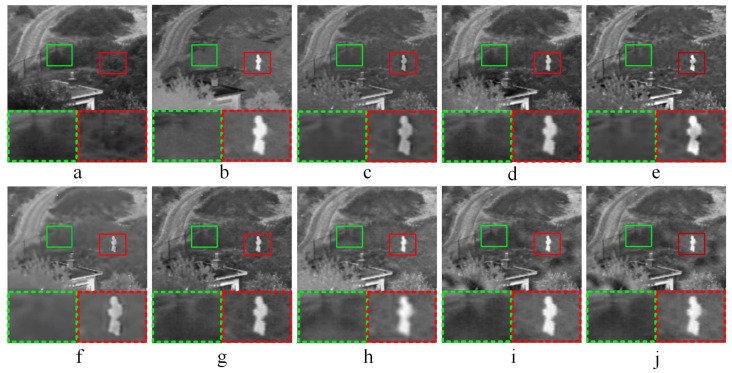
Infrared-visible image fusion comparative experiments—3. (a,b) are source images, (c–j) are the fused results of ASR, CNN, CT, KIM, MST-SR, NSCT-PCNN, NSCT-PCand the proposed method, respectively. At the bottom of each image, two areas marked in green and red dashed line frames correspond to the magnified areas encompassed in green and red frames, respectively.
